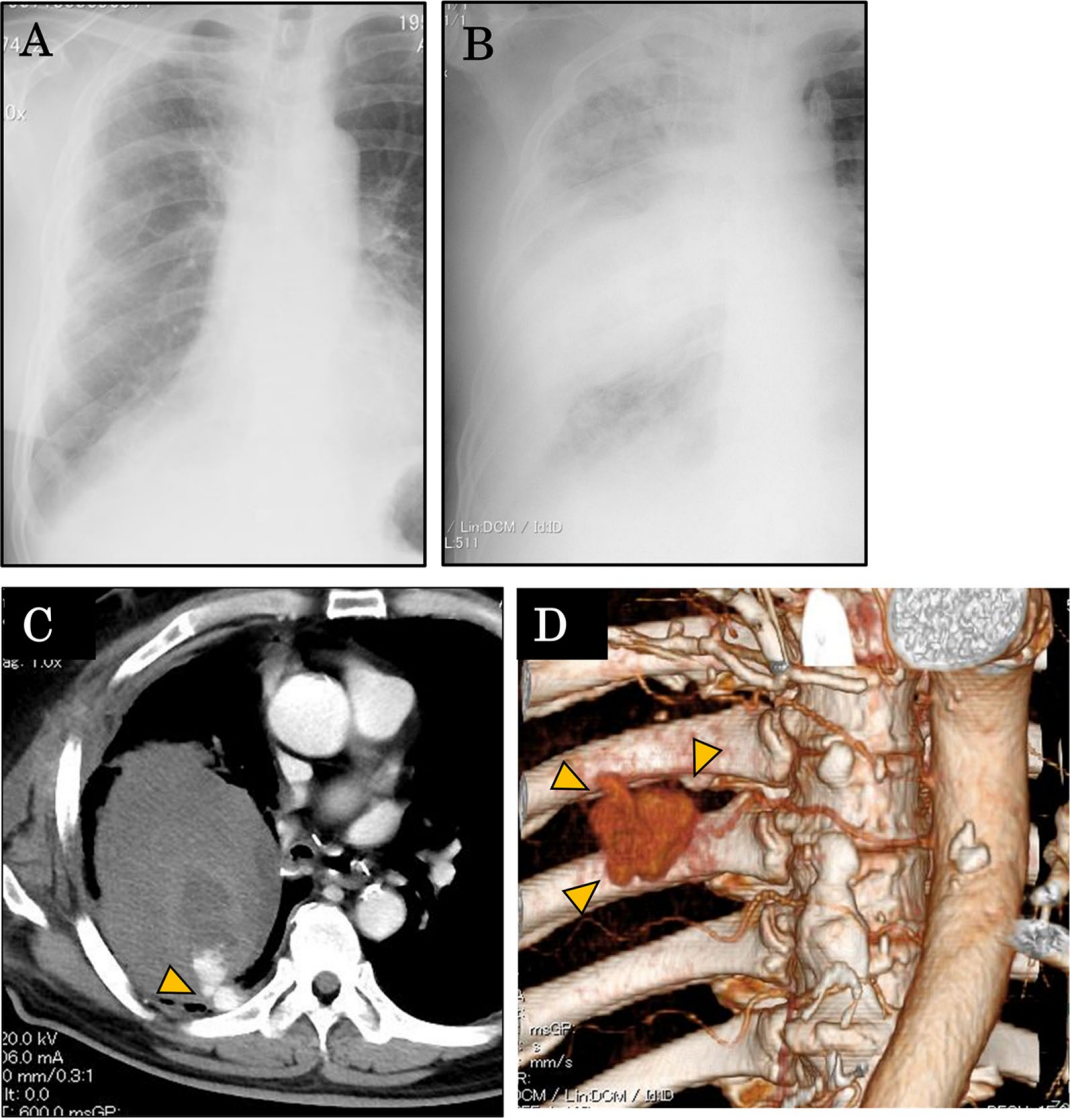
Radiological examinations performed on the day of late-onset bleeding (18 days after surgery). Chest X-rays before the bleeding (a) and after the emergency transportation (b) are shown. Contrast-enhanced CT examination revealed bleeding from the intercostal artery (arrowhead) and a large intrathoracic hematoma (c). The 3D reconstruction of the CT revealed a pseudoaneurysm (arrowhead) of the intercostal artery (d)
